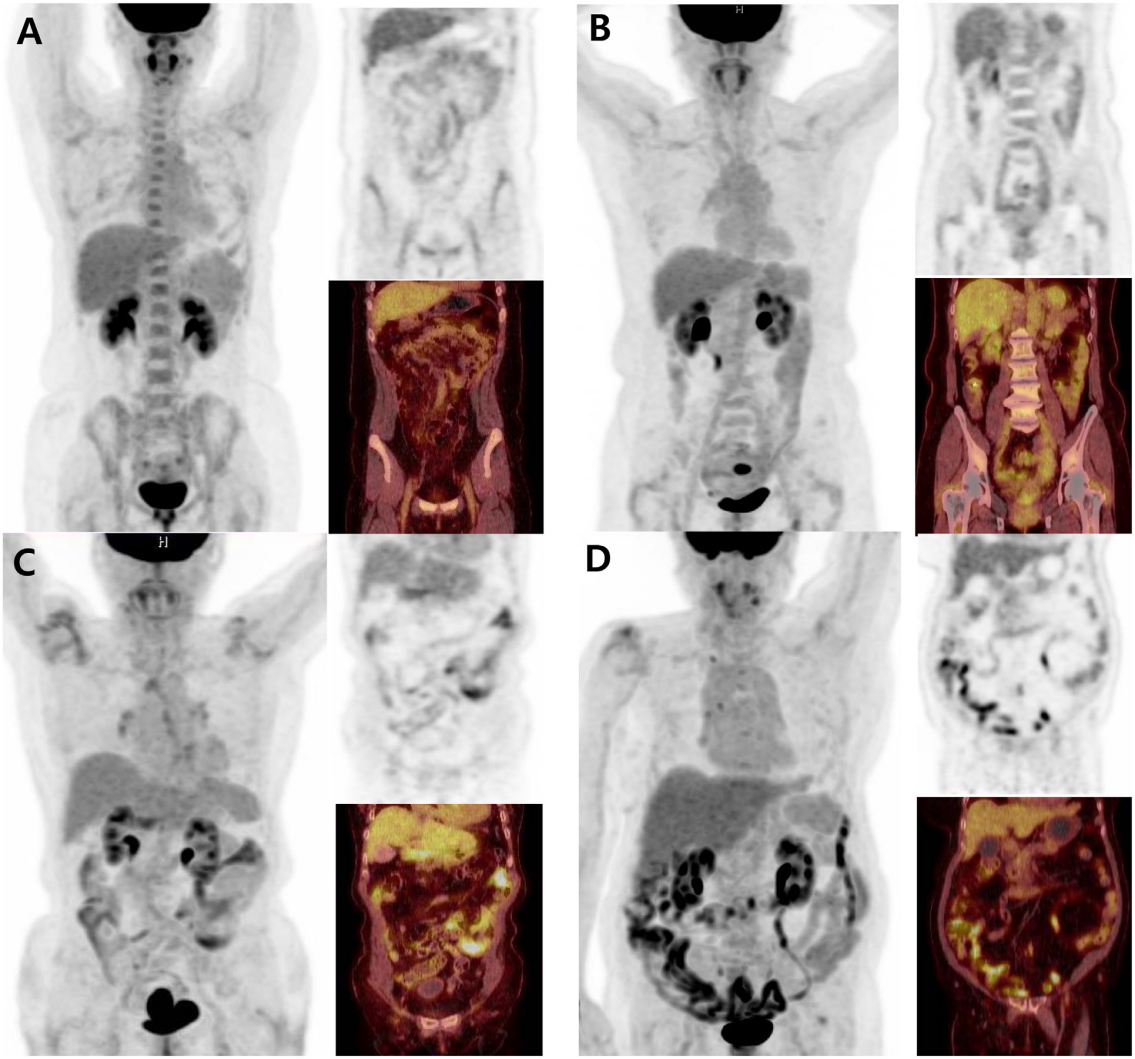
(A) The representative case of grade 1 background colonic ^18^F-FDG uptake on PET/CT scan (TB SUV_*max*_ of 1.9) is shown. (B) The representative case of grade 2 background colonic uptake on PET/CT scan (TB SUV_*max*_ of 2.0) is shown. (C) The representative case of grade 3 background colonic uptake on PET/CT scan (TB SUV_*max*_ of 3.4) is shown. (D) The representative case of grade 4 background colonic uptake on PET/CT scan (TB SUV_*max*_ of 5.3) is shown.
